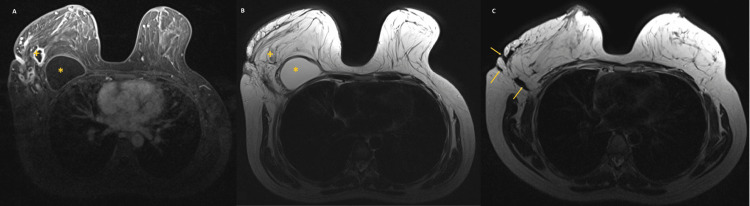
Breast MRI showing a collection (*) with thickened wall and gadolinium enhancement, several smaller abscesses (+), and fistulae (arrow) (A) T1-weighted. (B, C) T2-weighted MRI: magnetic resonance imaging
